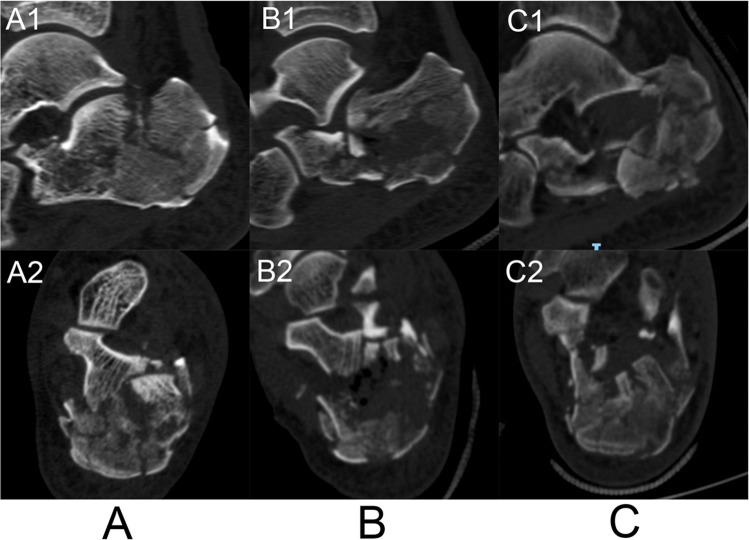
Comminuted tuberosity fragment. A–C Sanders types II, III, and IV, respectively. (A1, B1, C1) Sagittal CT scans. (A2, B2, C2) Coronal CT scans. CT, computed tomography
